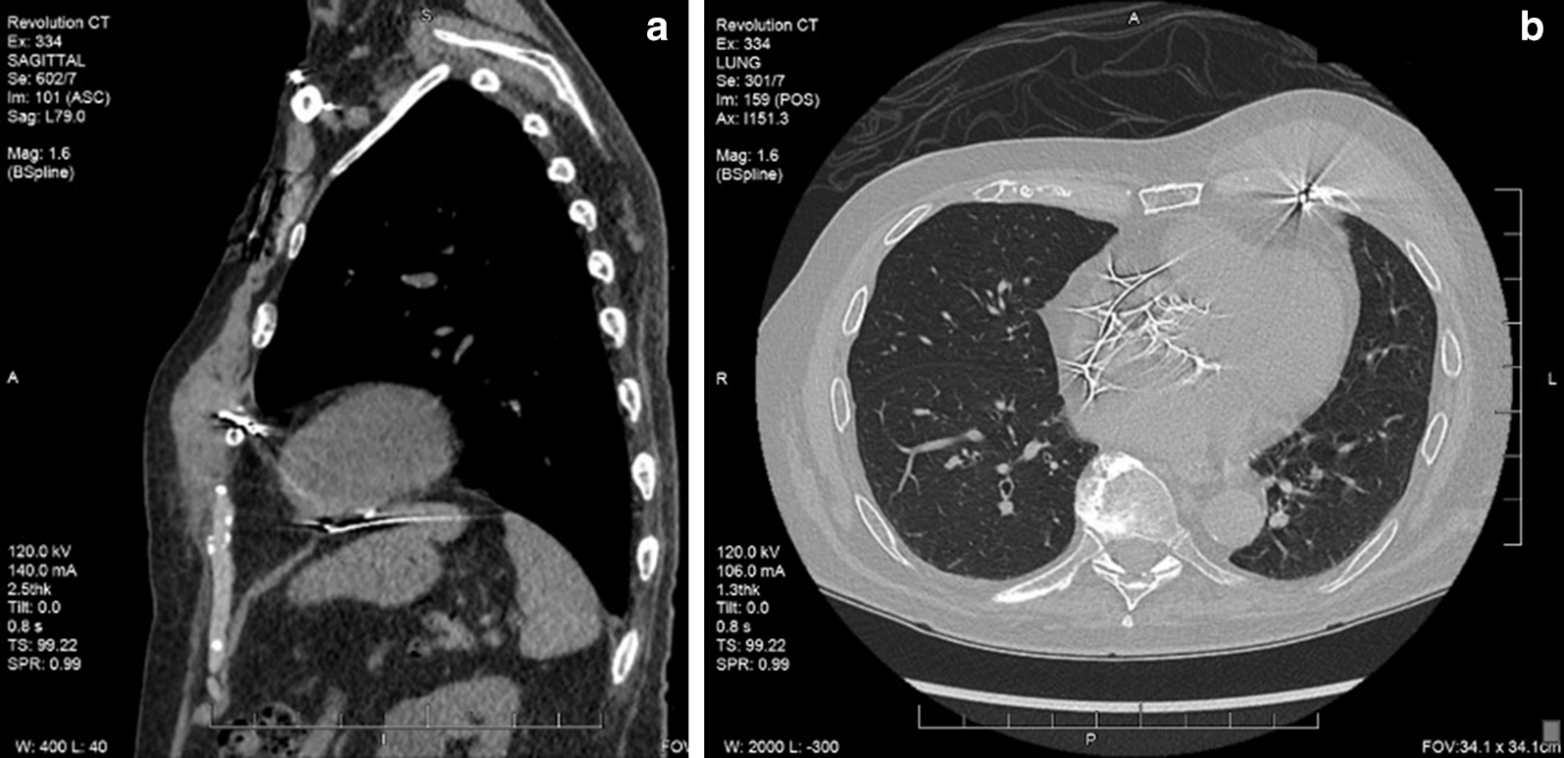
a Sagittal CT noting the ventricular lead rib perforation and anterior chest wall mass and b thoracic CT imaging the left anterior chest wall mass and erosion of ventricular pace lead into a rib
